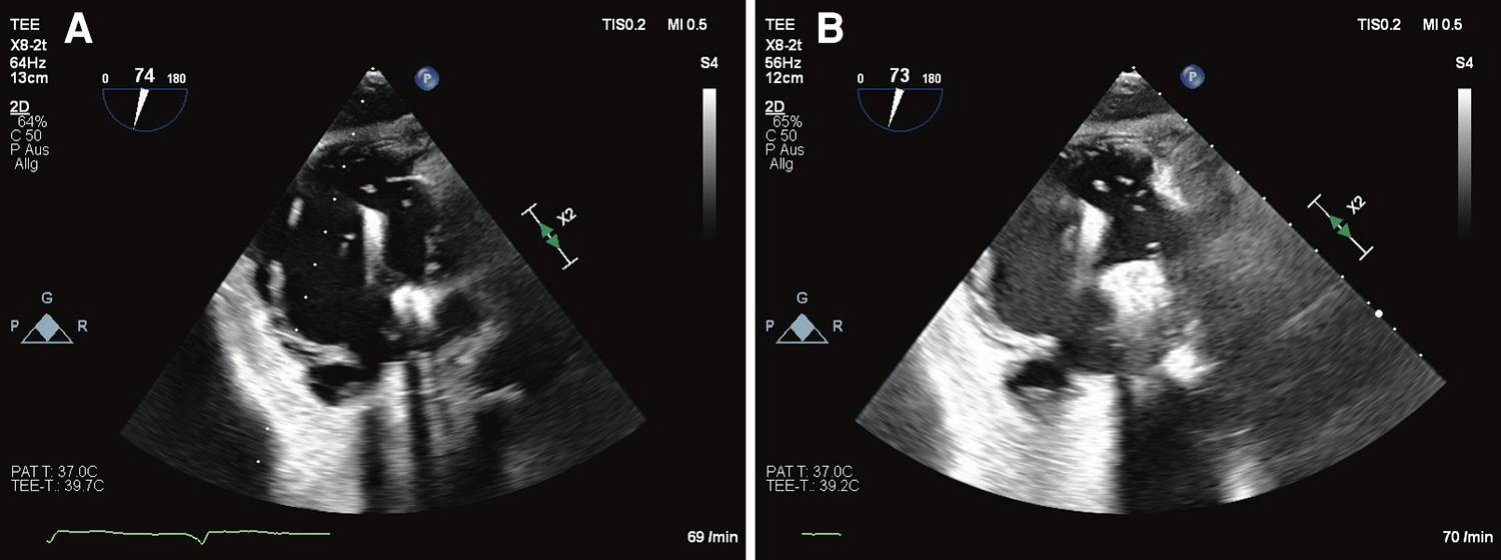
Procedural transoesophageal echocardiography showing the first implanted (*A*) and the second device (*B*) both in anteroseptal position in a transgastric short-axis view.
